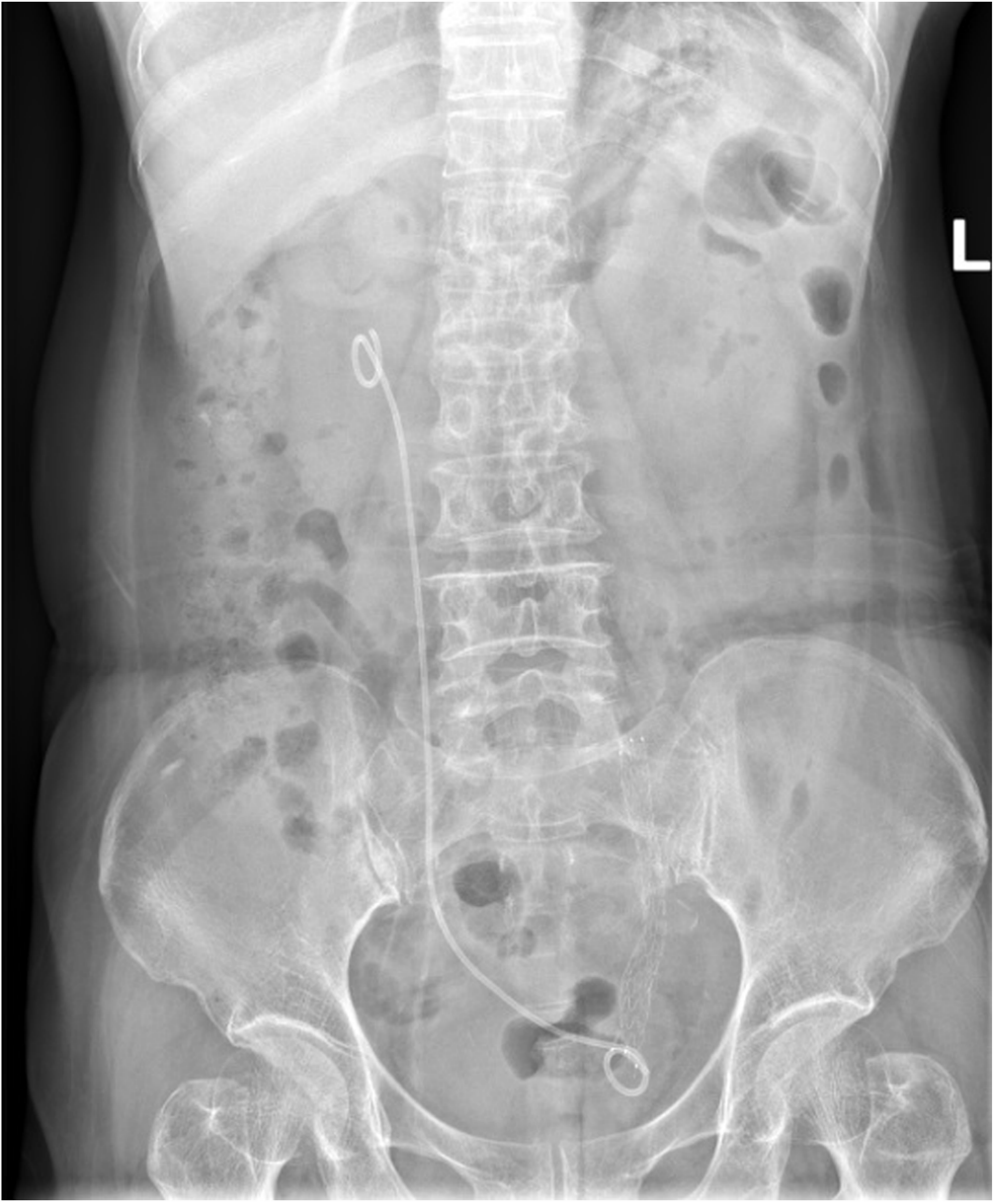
Kidney, ureter and bladder (KUB) radiography of Allium stent placement for repairing uretero‐vaginal fistula.
